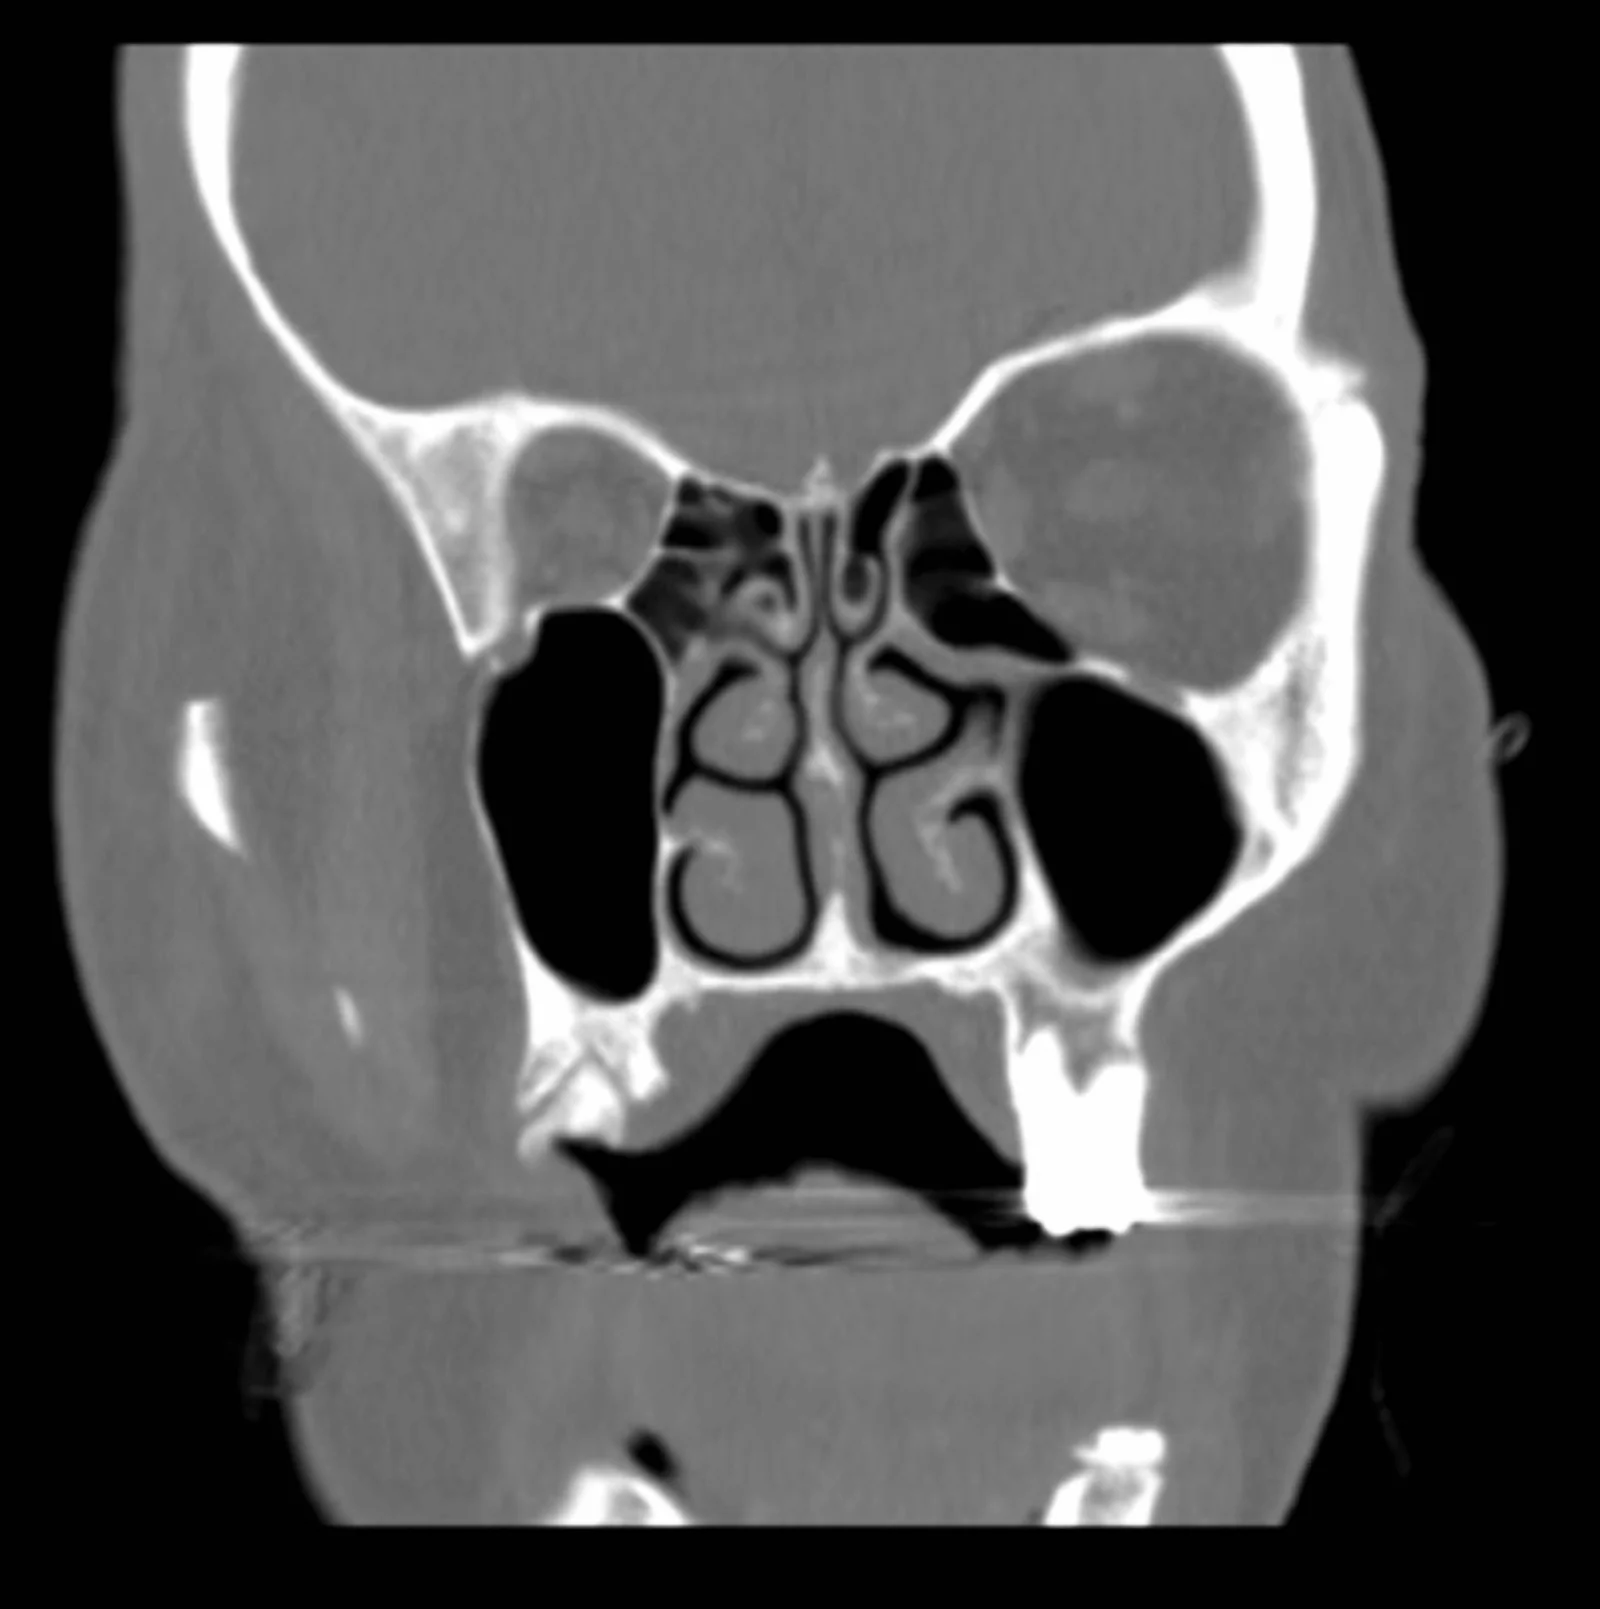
CT of the sinuses showing no evidence of infection. The absence of sinonasal disease supported a pulmonary presentation of mucormycosis without sinonasal involvement.
